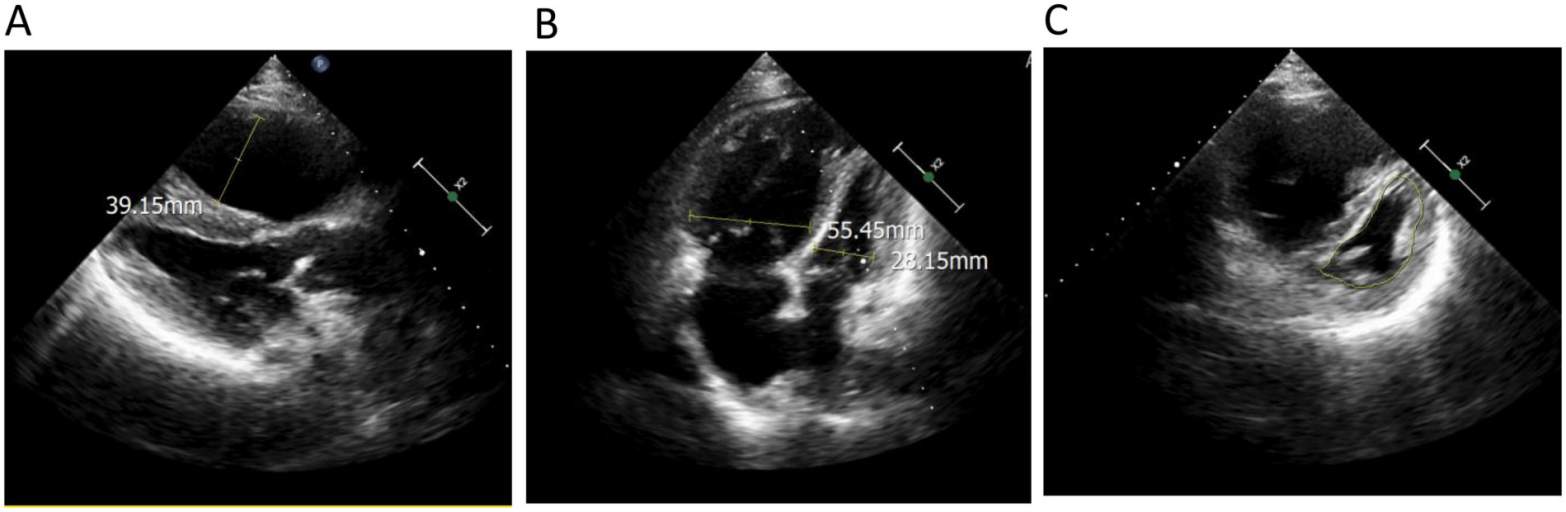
Echocardiography demonstrated (A) an enlarged right ventricle (right ventricular anteroposterior diameter 39.15 mm); (B) the ratio of right ventricular transverse diameter to left ventricular transverse diameter was significantly higher than 1 (right ventricular transverse diameter 55.45 mm, left ventricular transverse diameter 28.15 mm); (C) the left ventricle showed a ‘D’-shaped configuration (indicated by the yellow line) due to compression.
